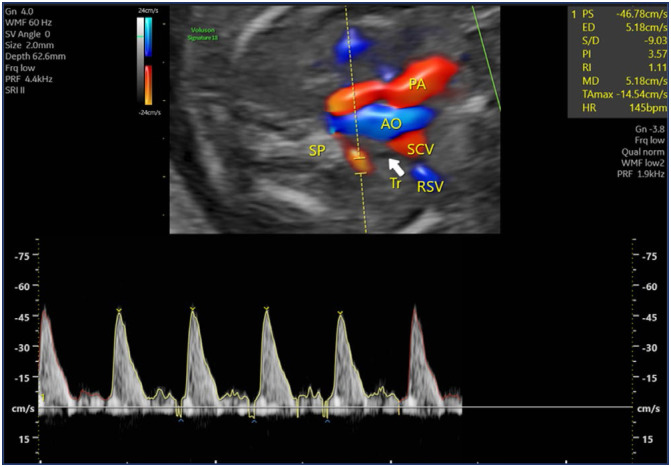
Color Doppler image of the aberrant right subclavian artery (ARSA)
